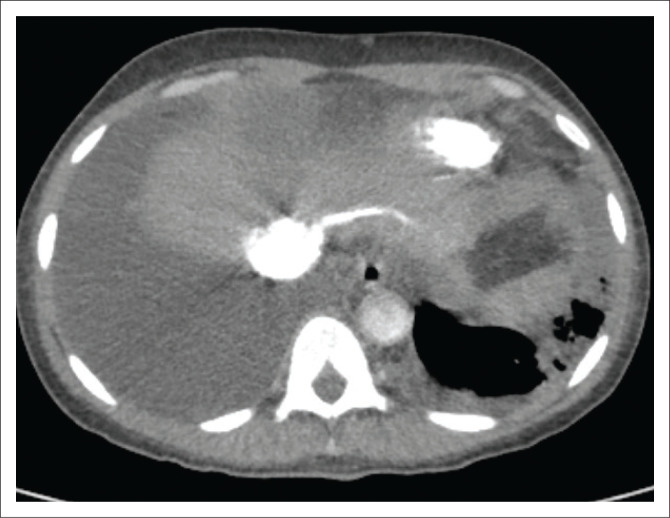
Axial CT of a patient with a large right and smaller left pleural effusion.
